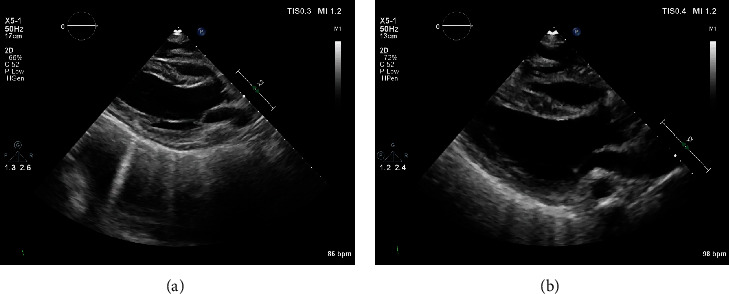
(a–b) Transthoracic echocardiogram demonstrating parasternal long axis view demonstrating hypokinesis of basal left ventricle and akinesis in all other segments (see supplementary material for video of echocardiogram clips in Figures 1–3). (b) Parasternal long axis view demonstrating recovery.
